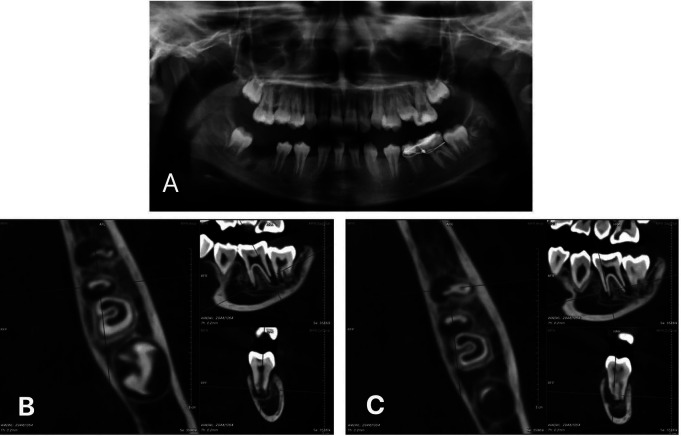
(a) Preoperative panoramic radiograph of tooth #36. (b) Preoperative CBCT scan with the slice through the distal root. (c) Preoperative CBCT scan with the slice through the mesial root.
